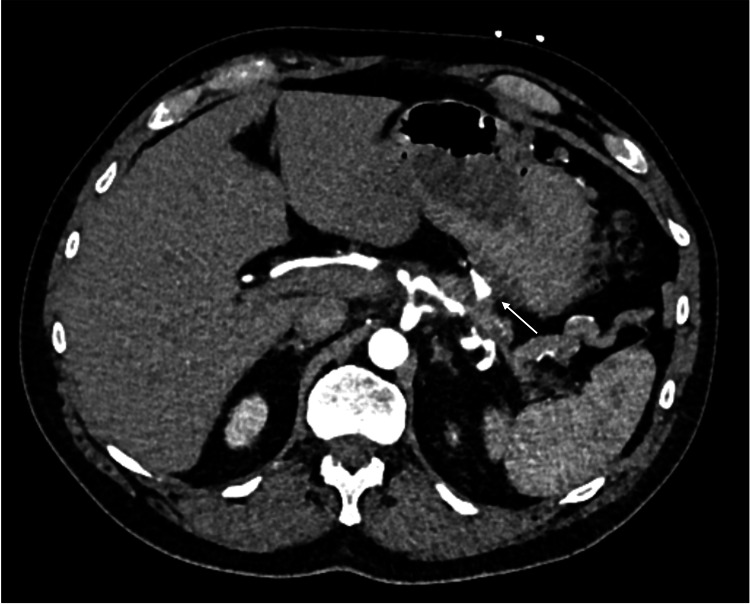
CT angiogram (transverse plane) showing left gastric artery territory pseudoaneurysm
